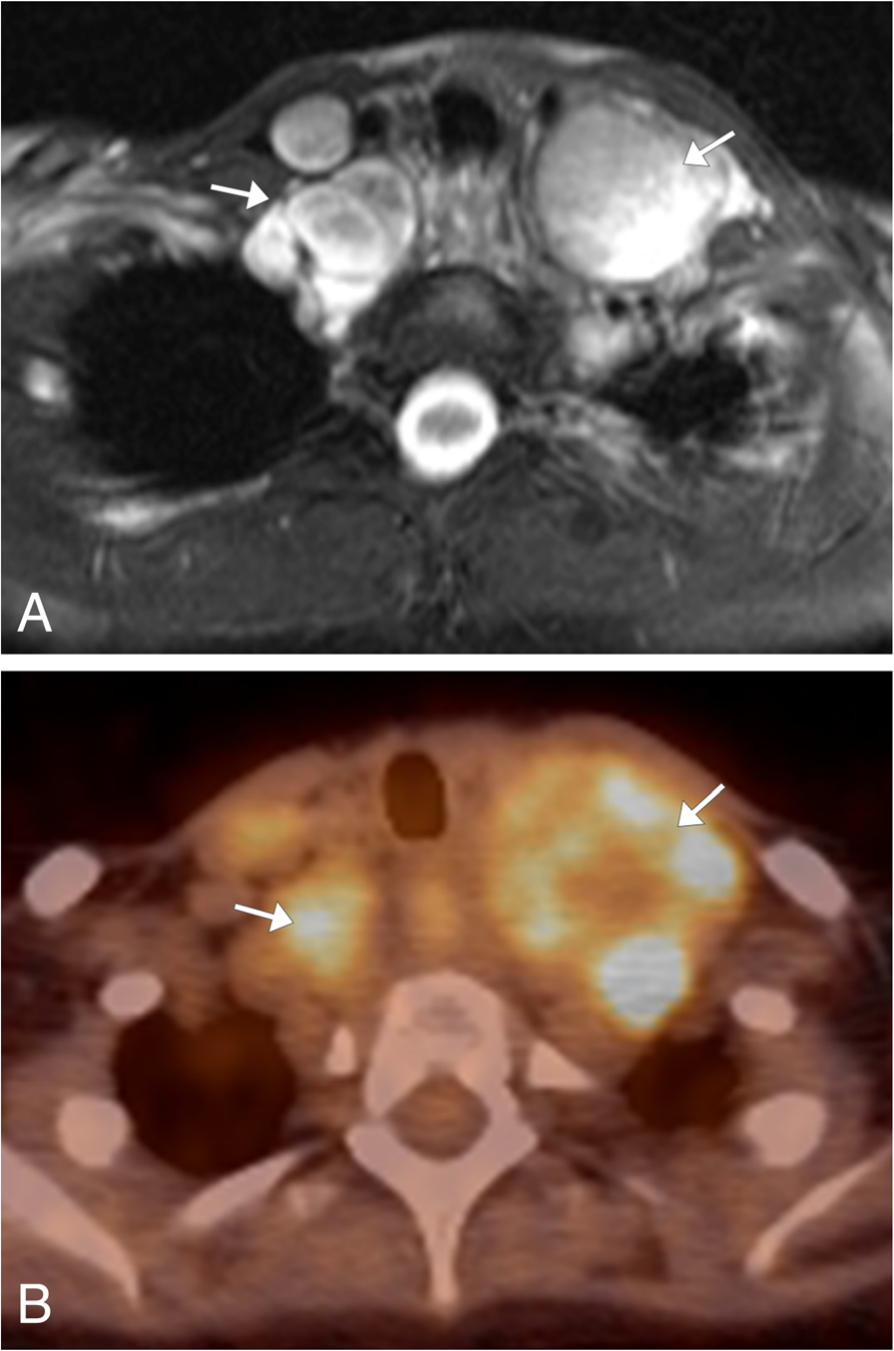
Malignant peripheral neural sheath tumor (MPNST). a Axial T2WI MR of the neck base demonstrating several round well-circumscribed large lesions which are predominantly hyperintense (arrows). The classic target sign on T2WI is lost. FDG-PET/CT axial (b) image shows these lesions to have heterogeneous increased metabolic activity (arrows)
